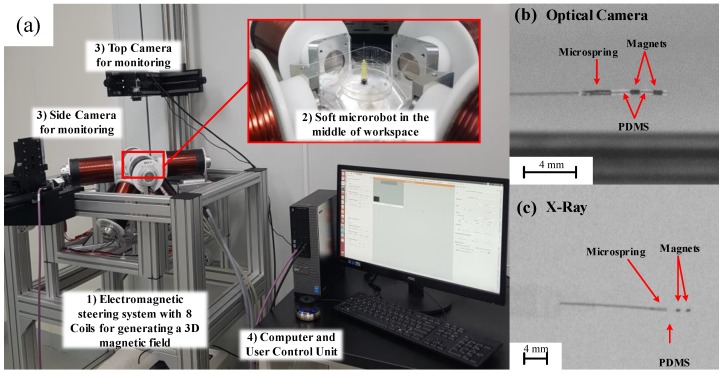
(a) The experimental setup for microrobot steering with the OctoMag system, (b) Optical image of the microrobot, (c) X-ray image of the microrobot.
